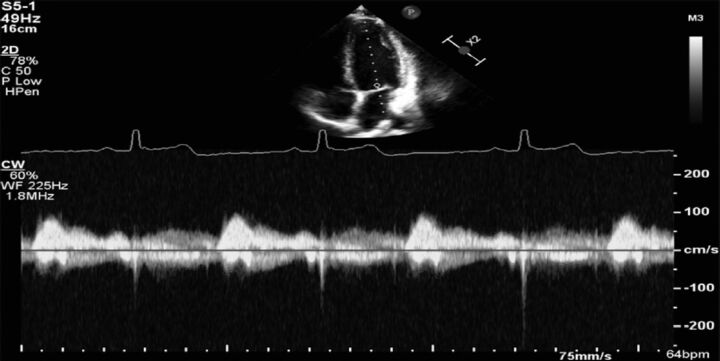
Echocardiography on the first day of admission; left ventricle size and wall thickness.
